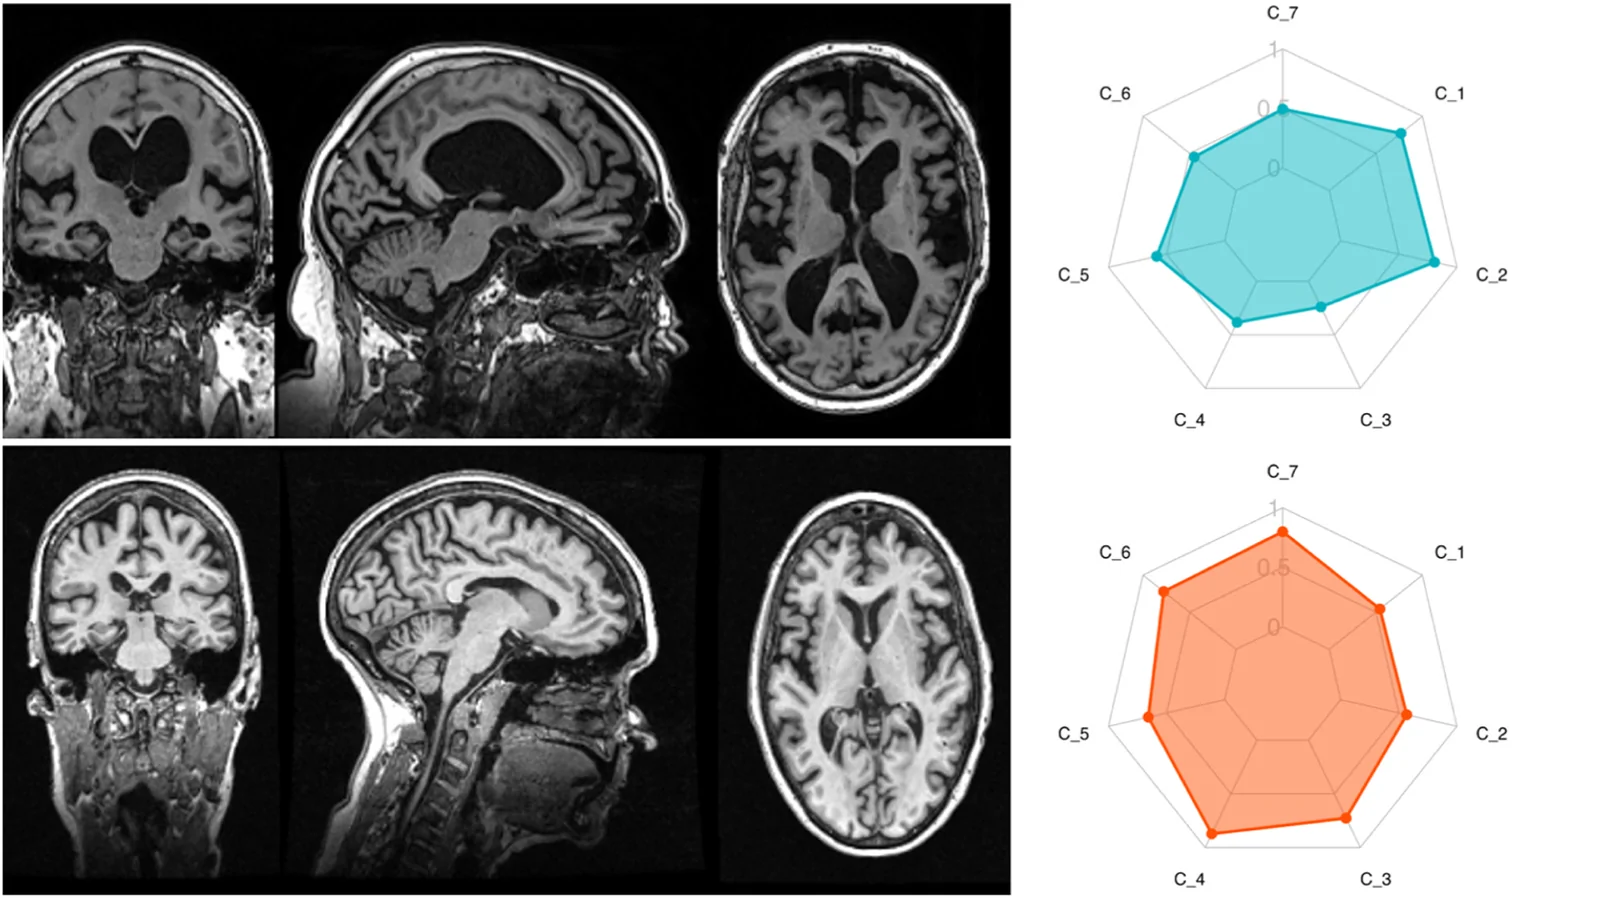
Example of 7 components’ loads in two individuals with opposite radiographic phenotype. The top case has iNPH with a DESH phenotype and loaded high on components 1 and 2 (high probability of CSF in the sylvian fissures and infra-sylvian sulci; low probability of CSF at the vertex) and low on 3, 4, and 6. The bottom case is a healthy control with no NPH features and loaded higher on 4, 6, and 7 and low on 1 and 2 (high probability CSF in the supra-sylvian sulci).
